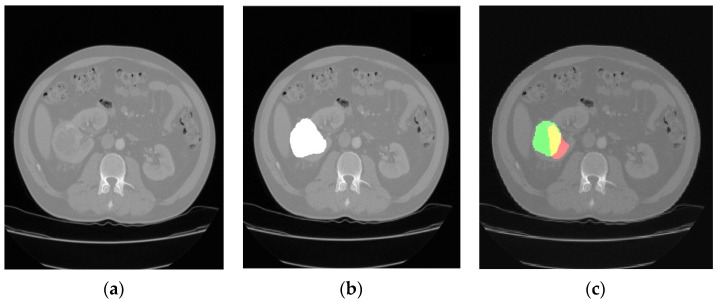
CT image of a kidney with clear cell renal cell carcinoma (a); the medical expert tumor segmentation (shown in white) (b); and the result of the tumor location prediction (c).
